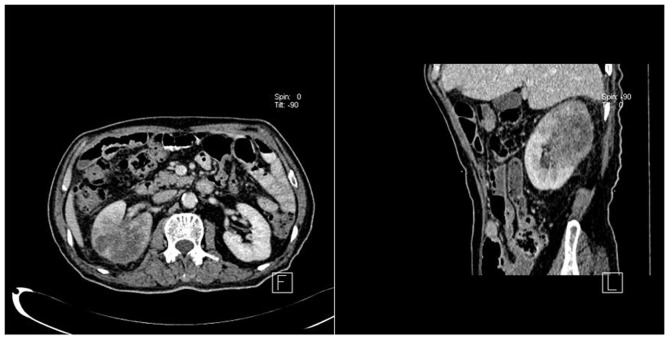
Abdominal computed tomography scan showing a large mass in the upper-mid portion of the right kidney with mixed density.
